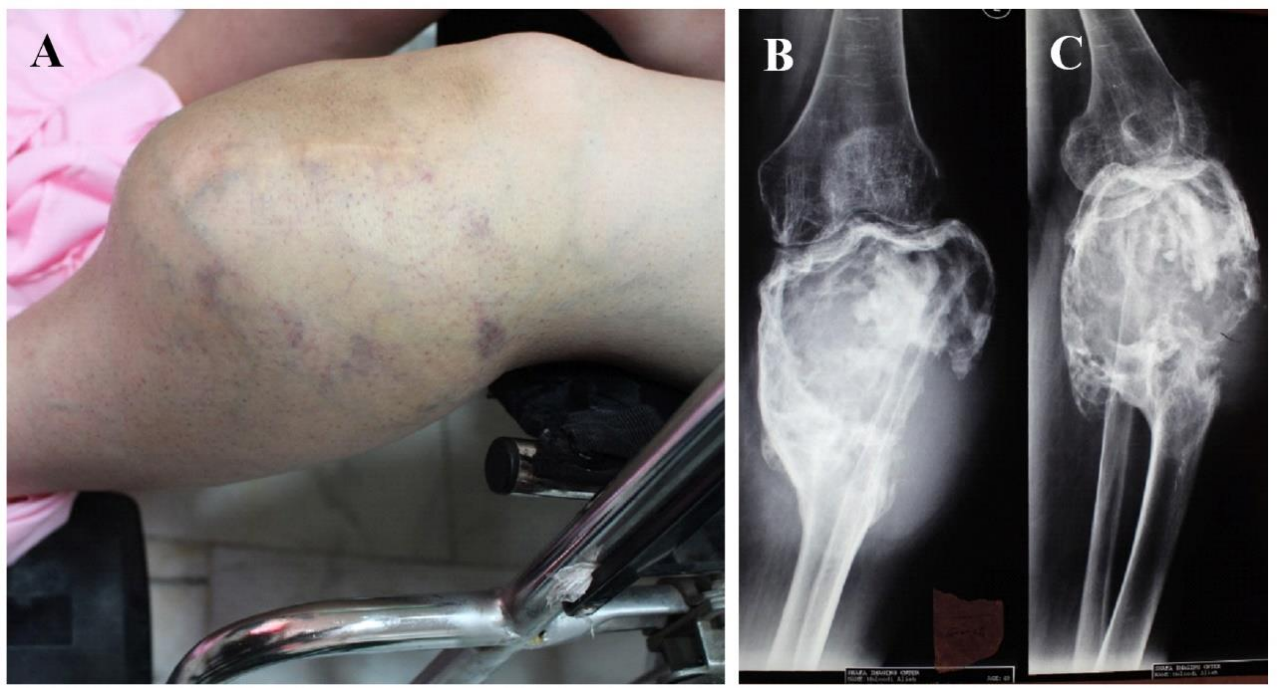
Neglected large mass below the knee 10 years after recurrence (A), plain radiographs revealed a large expansile destructive bony lesion containing mixed areas of lytic and sclerotic components associated with large soft tissue mass (B & C)
